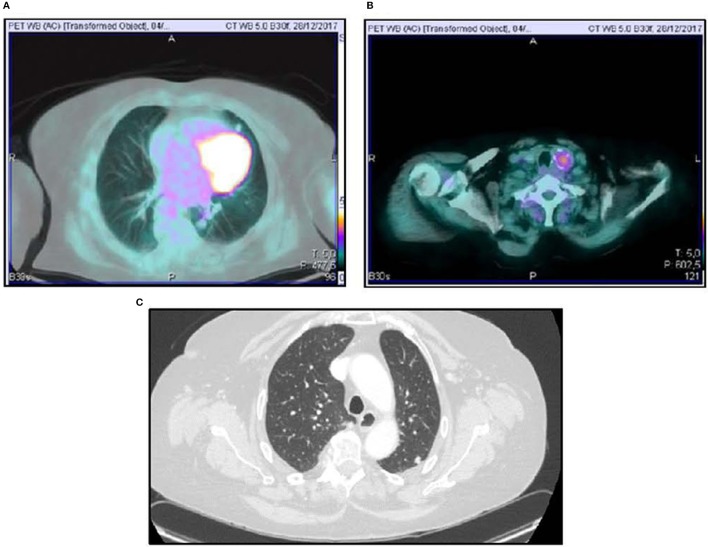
18FDG-PET scan showing lung uptake (A) and thyroid uptake (B). Computed Tomography Imaging of lung metastasis (C).
